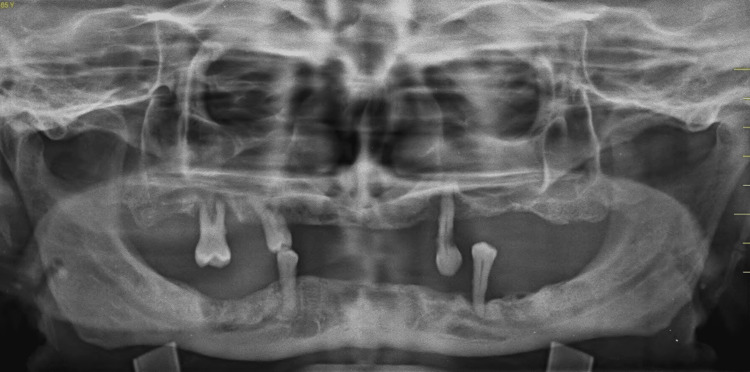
Orthopantomogram of the patient showing resorbed ridges and insufficient bone height
